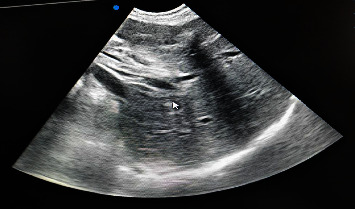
Ultrasound image of ascaris worm in the common bile duct, vertical section.
